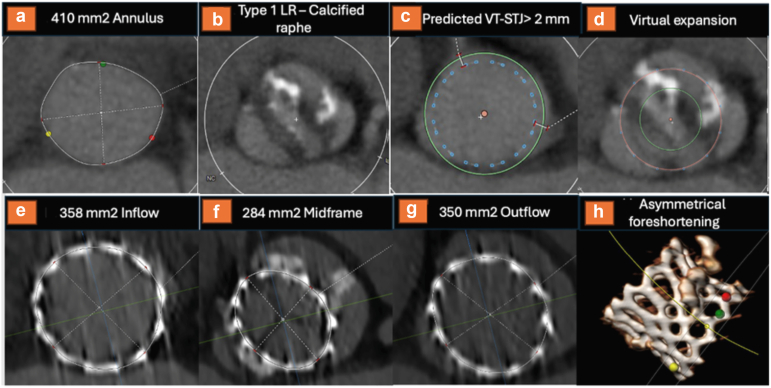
CT-based mechanistic assessment of late structural valve deterioration after TAVR in bicuspid anatomy. A 62-year-old woman with severe aortic stenosis and acute decompensated heart failure (left ventricular ejection fraction, 20%) underwent urgent TAVR, with recovery of ejection fraction to 56%. At 5-year follow-up, she developed VARC-3 structural valve deterioration with elevated transvalvular gradients and severe patient-prosthesis mismatch. Preprocedural CT showed (a) an annular area of 410 mm^2^, (b) bicuspid aortic valve (Sievers type 1, left-right fusion) with calcified raphe, (c) predicted valve-to-sinotubular junction distance >2 mm, and (d) software-derived virtual expansion of a nominal 23-mm balloon-expandable valve (Sapien platform). In the context of heavy raphe calcification, this anatomy suggested a likely constraint to full frame expansion. Follow-up CT showed (e) preserved inflow expansion (358 mm^2^), (f) severe midframe underexpansion (284 mm^2^) with marked eccentricity, (g) preserved outflow expansion (350 mm^2^), and (h) volume-rendered reconstruction demonstrating asymmetric foreshortening and marked frame underexpansion. These findings provide a mechanistic explanation for late structural valve deterioration and limit the feasibility of redo transcatheter valve-in- valve intervention. She was referred for elective surgical explantation and surgical bioprosthetic valve replacement Abbreviation: CT, computed tomography; LR, left and right coronary cusps; TAVR, transcatheter aortic valve replacement; VARC, Valve Academic Research Consortium; VT-STJ, valve-to-sinotubular junction distance.
